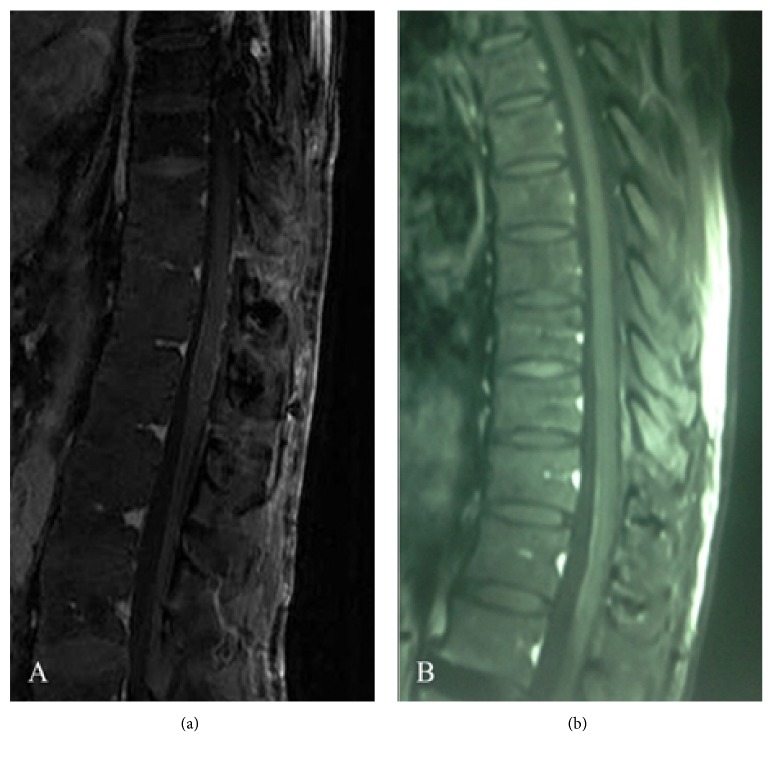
(a) T1-weighted sagittal MRI after surgery and (b) at a 6-month follow-up showing no evidence of a mass.
